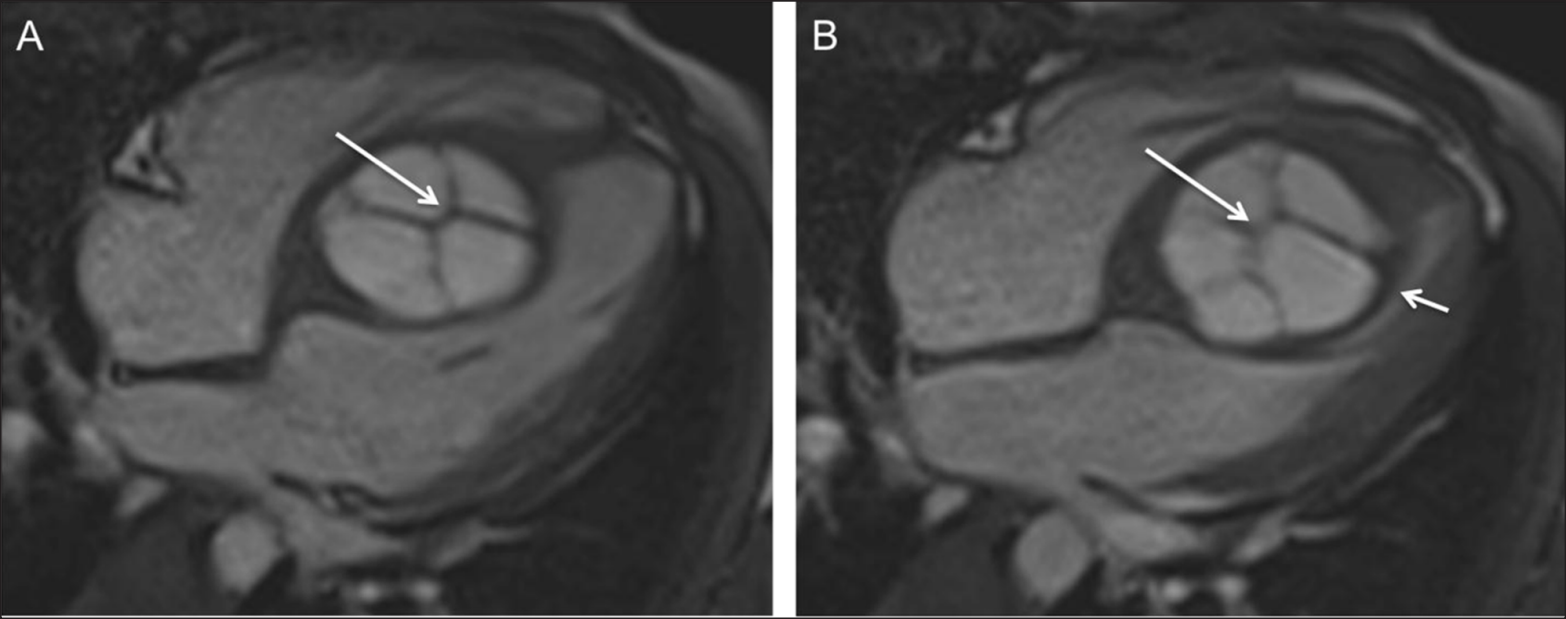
The four chamber view images of the patient on steady-state free precision MRI during the diastole (a) and the systole (b). The lesion has hyperintense signal with internal septations. Also, note that the mid-ventricular and apical part of the left ventricular cavity is substantially obliterated by the mass during the systole (short arrow).
